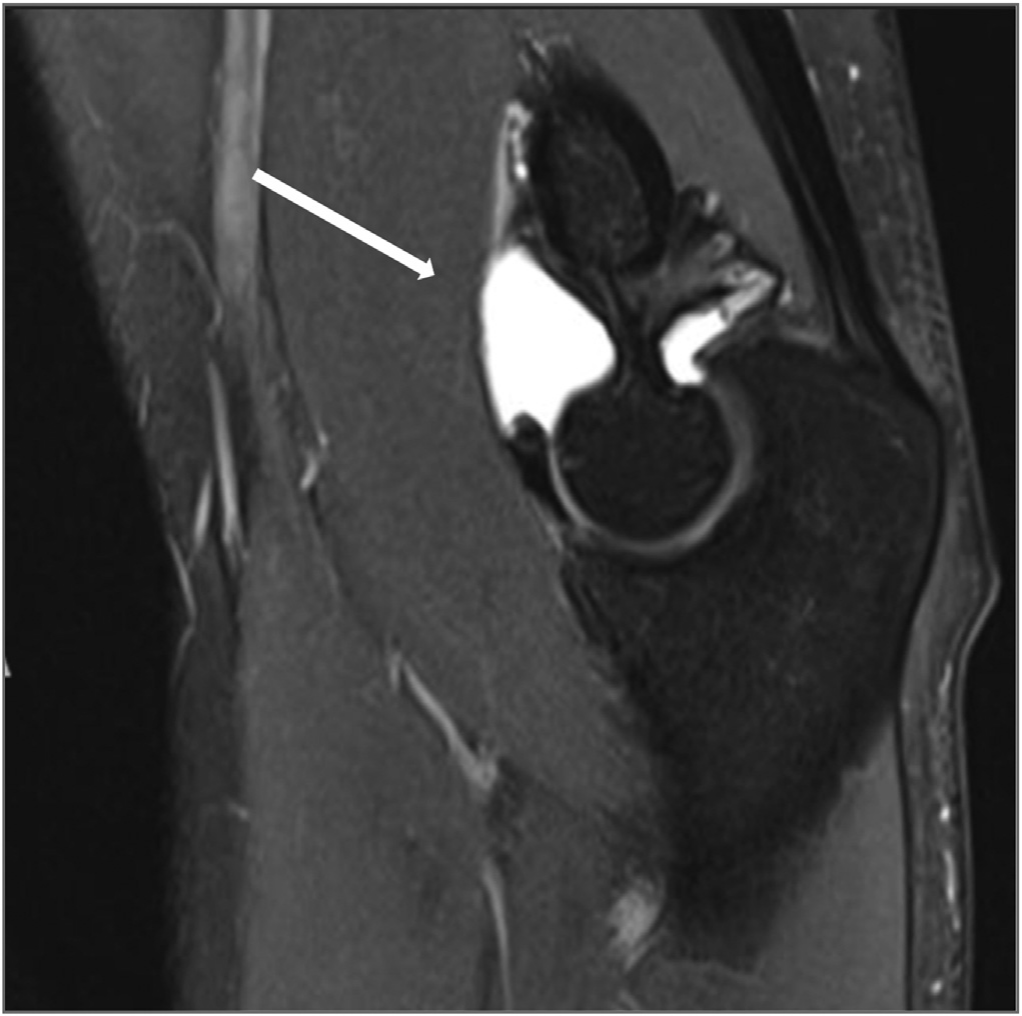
Postoperative MRI depicting clear elbow joint space (white arrow) and absence of synovial thickening. *MRI*, magnetic resonance imaging.
